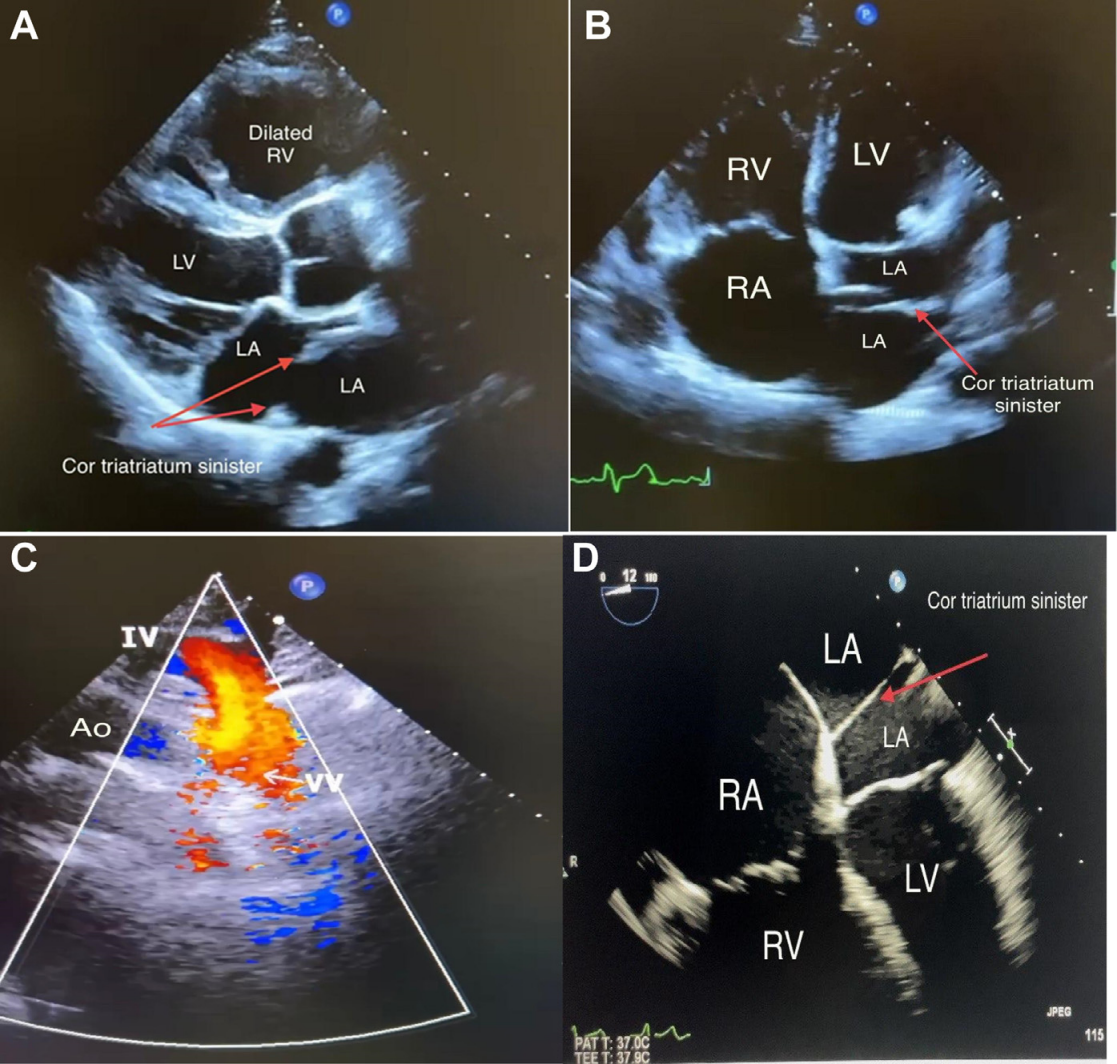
Transthoracic and Transesophageal Echocardiography (A, B, D) Significantly dilated RV and cor triatriatum sinister is shown: a thin fibromuscular membrane found inside the LA that causes division of the LA into 2 parts (red arrows). (C) VV: suprasternal view at the short axis of the aortic arch—a dilated vessel with a red color flow on color Doppler study suggestive of a venous structure with blood drainage into the IV. Ao = Aorta; IV = innominate vein; LA = left atrium; LV = left ventricle; RA = right atrium; RV = right ventricle; VV = vertical vein.
